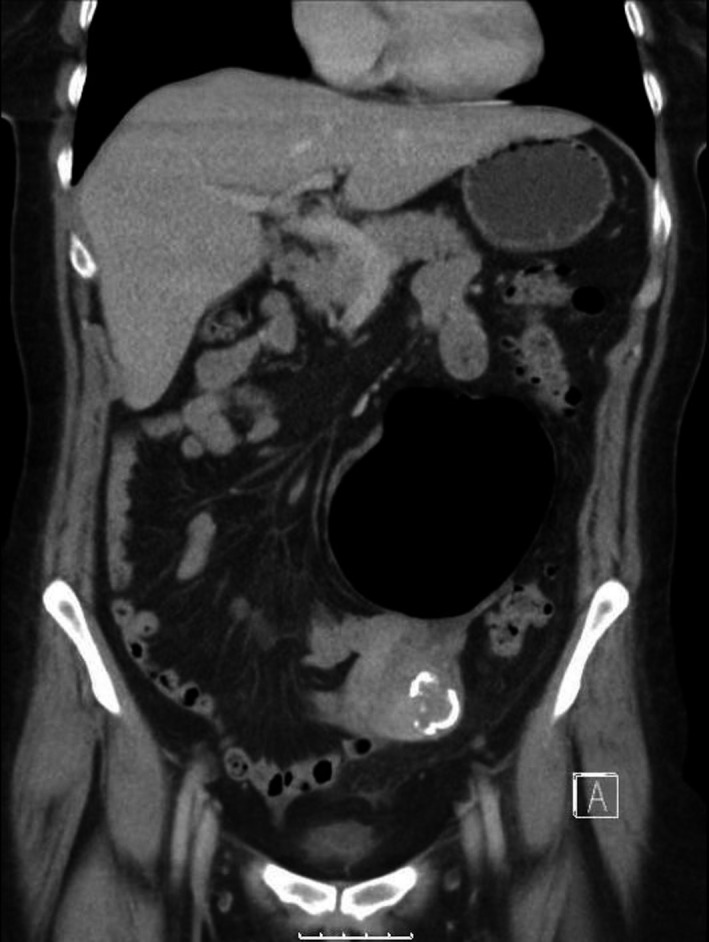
CT image (coronal view).
